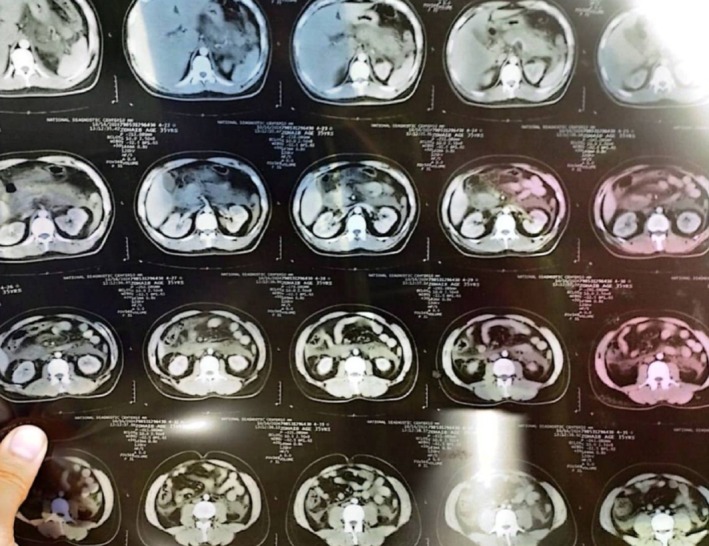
Pelvic CT Sections Confirming the absence of obstructive masses and evaluating the extent of free fluid within the pelvic cavity.
